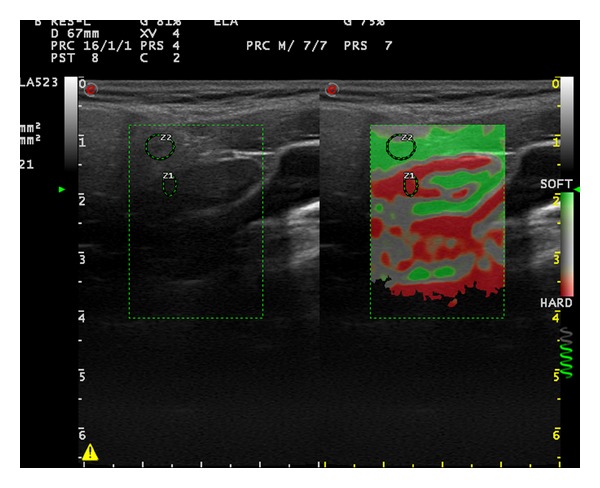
Elastosonography of the cervical lymph node on the left side of a 50-year-old female patient (long axis: 24.6 mm, short axis: 7.7 mm, cortical thickness: 2.6 mm, hilar thickness: 4.9 mm, strain ratio: 38.6, and elasticity score: 4).
